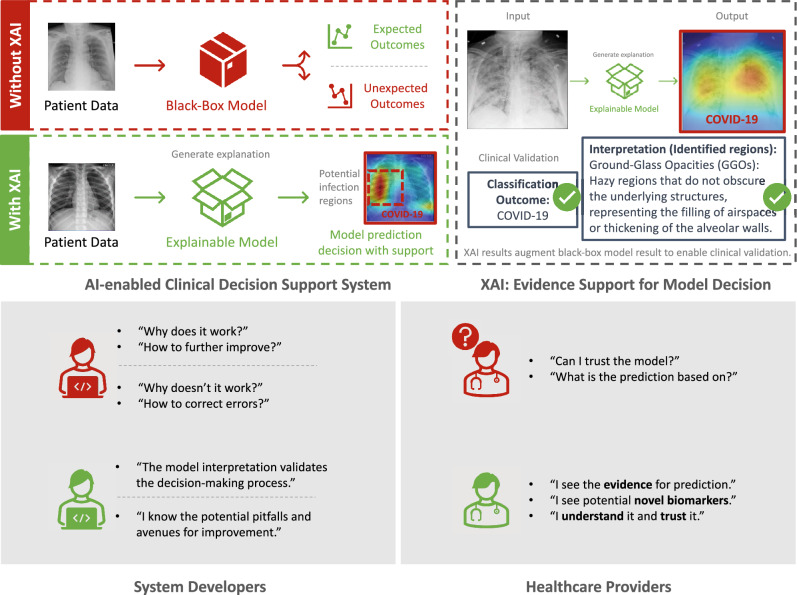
Motivation for model developers and clinicians to implement XAI in AI-enabled clinical decision support systems. XAI approaches imbue originally opaque, black-box models with the ability to pinpoint or emphasize areas that contribute most significantly to the final decision-making process. For example, for a case study in COVID-19 diagnosis utilizing X-ray imaging, XAI could augment original black-box model (decision only) by highlighting potential infectious regions like Ground-Glass Opacities (GGOs), which are indistinct regions that do not mask the underlying structures and typically signify the filling of airspaces or thickening of alveolar walls^56^. For healthcare system developers, such interpretation enables the validation of the decision-making process and the identification of potential pitfalls for improved model performance. For healthcare providers as end-users, XAI provides evidence of prediction and facilitates the exploration of potential novel biomarkers for clinical confidence and widespread adoption.
